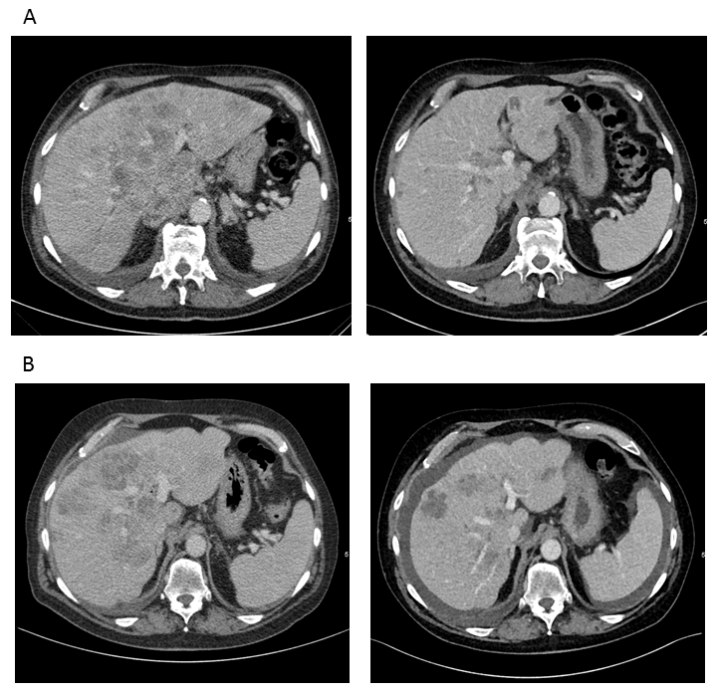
CT-scans reveal a marked response to therapy until the fourth line of treatment (patient 1) A. CT scan before (left) and after (right) induction of first line poly-chemotherapy according to FOLFOX6 protocol. B. CT scan before (left) and after (right) 4th line chemotherapy according to FOLFIRI protocol. According to RECIST criteria, a partial response with -36 % calculated for all target lesions was achieved.
